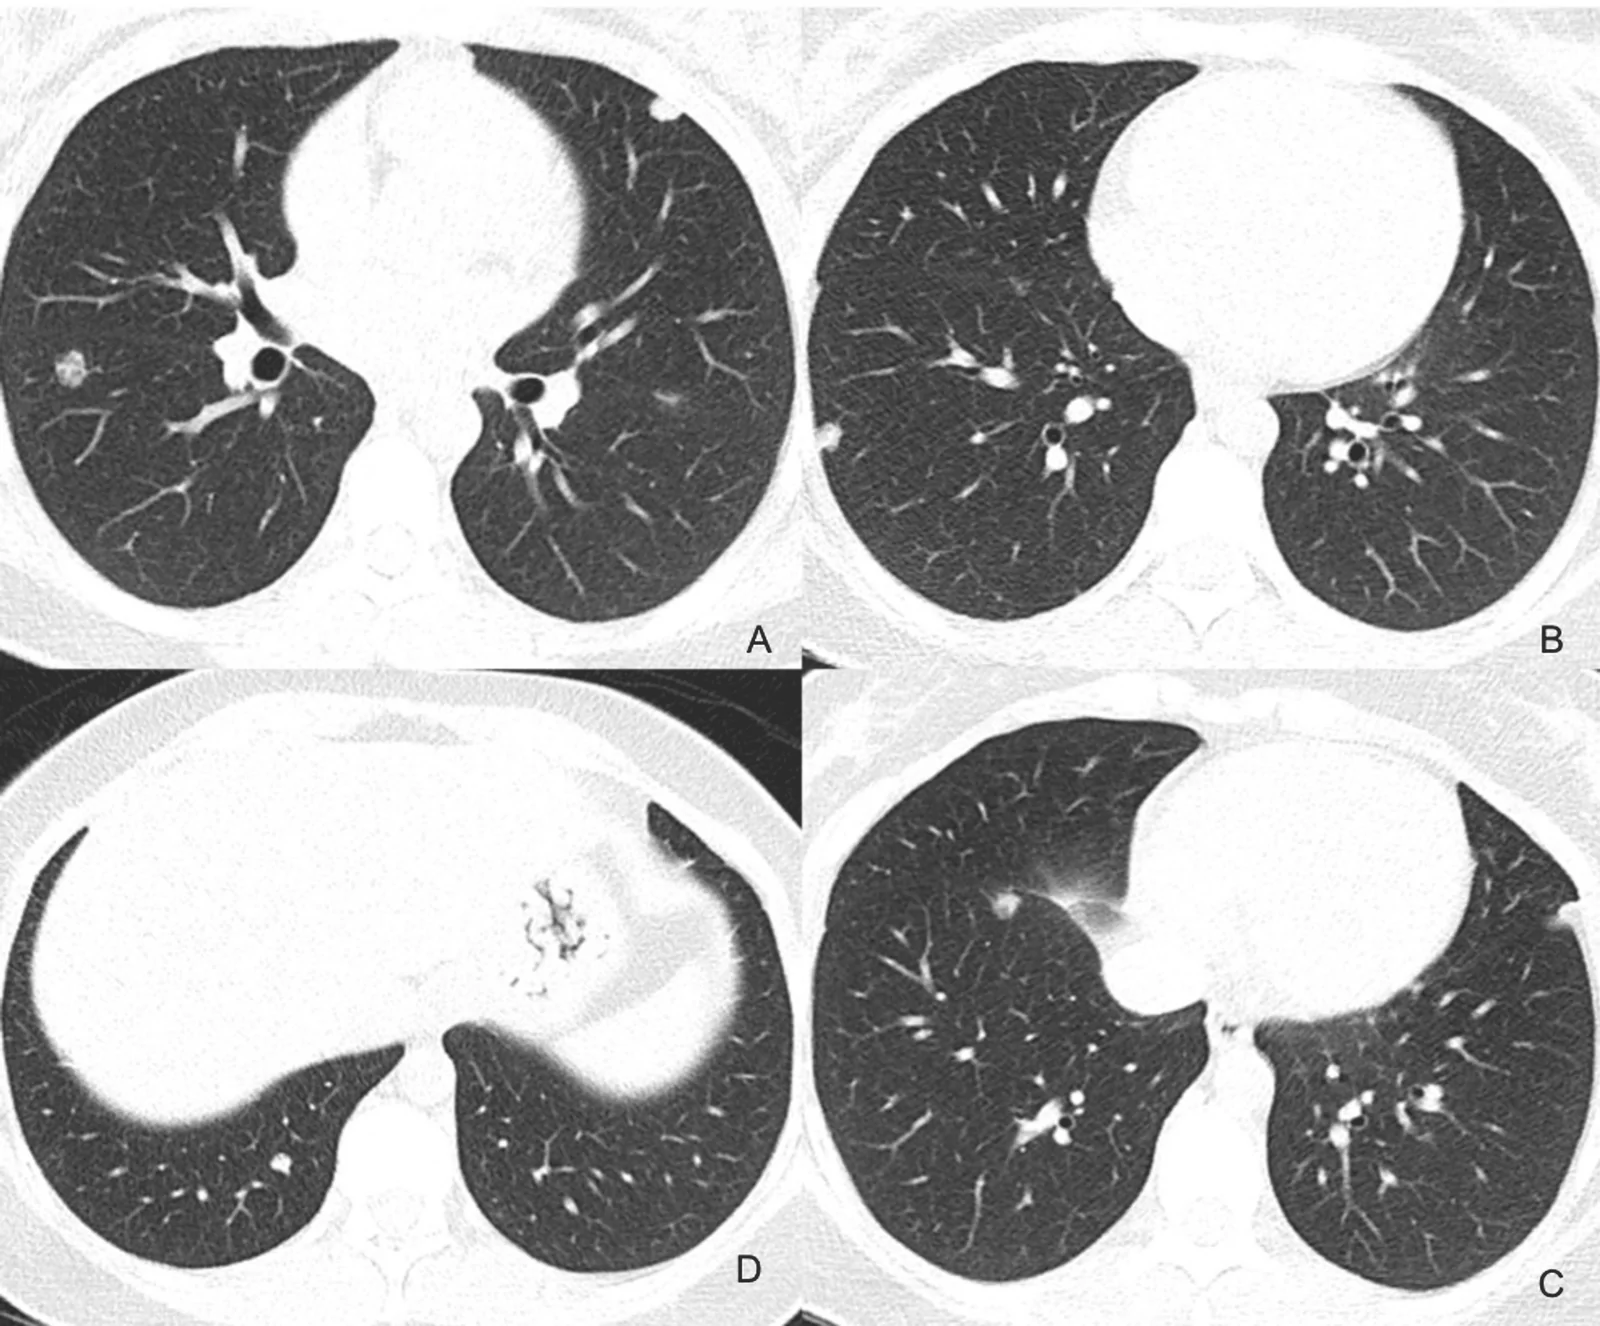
CT of the thorax demonstrating multiple lung metastases. Axial CT images of the thorax at four different levels, arranged clockwise from the most superior level (A) to the most inferior level (D), demonstrate multiple bilateral pulmonary nodules consistent with metastases.
